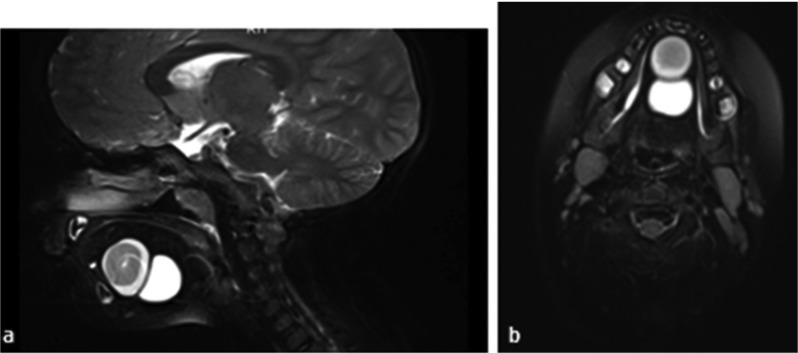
a: Sagittal (Magnetic resonance imaging) MRI scan showing the relationship between the two lesions in the tongue and on the ﬂoor of the mouth. b: Coronal Magnetic resonance imaging (MRI) scanned image of the two lesions.
